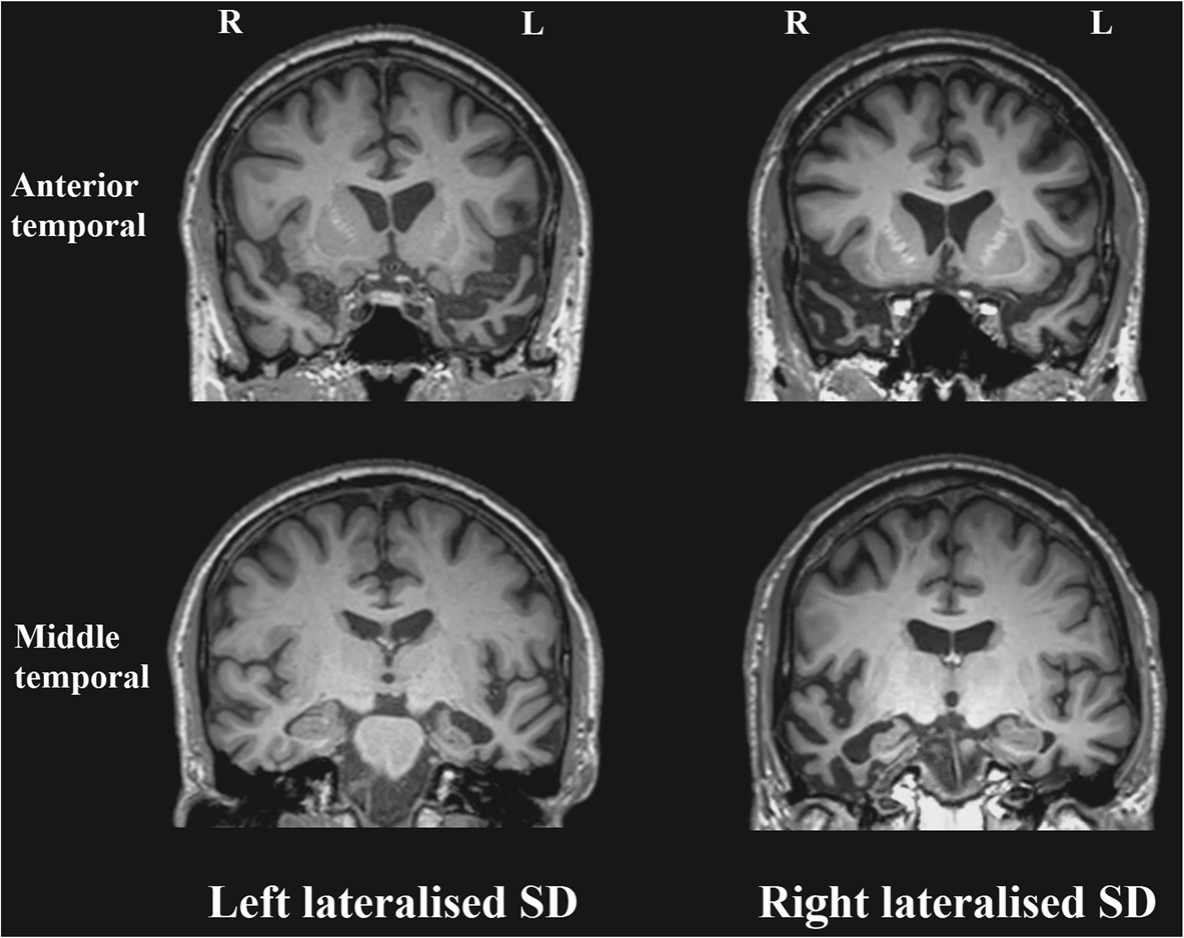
Axial MRI scans showing typical anterior and middle temporal structural abnormalities in early left and right lateralised SD. *L* left, *R* right, *SD* semantic dementia
